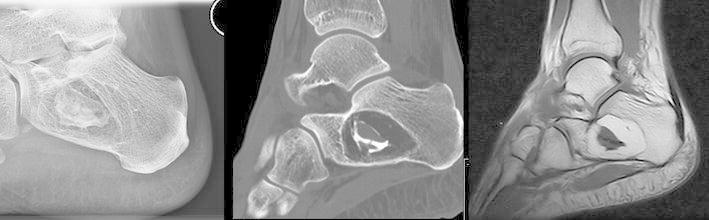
Typical radiographic appearance of calcaneal lipoma of bone: plain radiography (*left*), sagittal CT-scan (*center*) and T1-weighted sagittal MRI (*right*) show an osteolytic lesion with central calcifications surrounded by fat-intense soft-tissue
